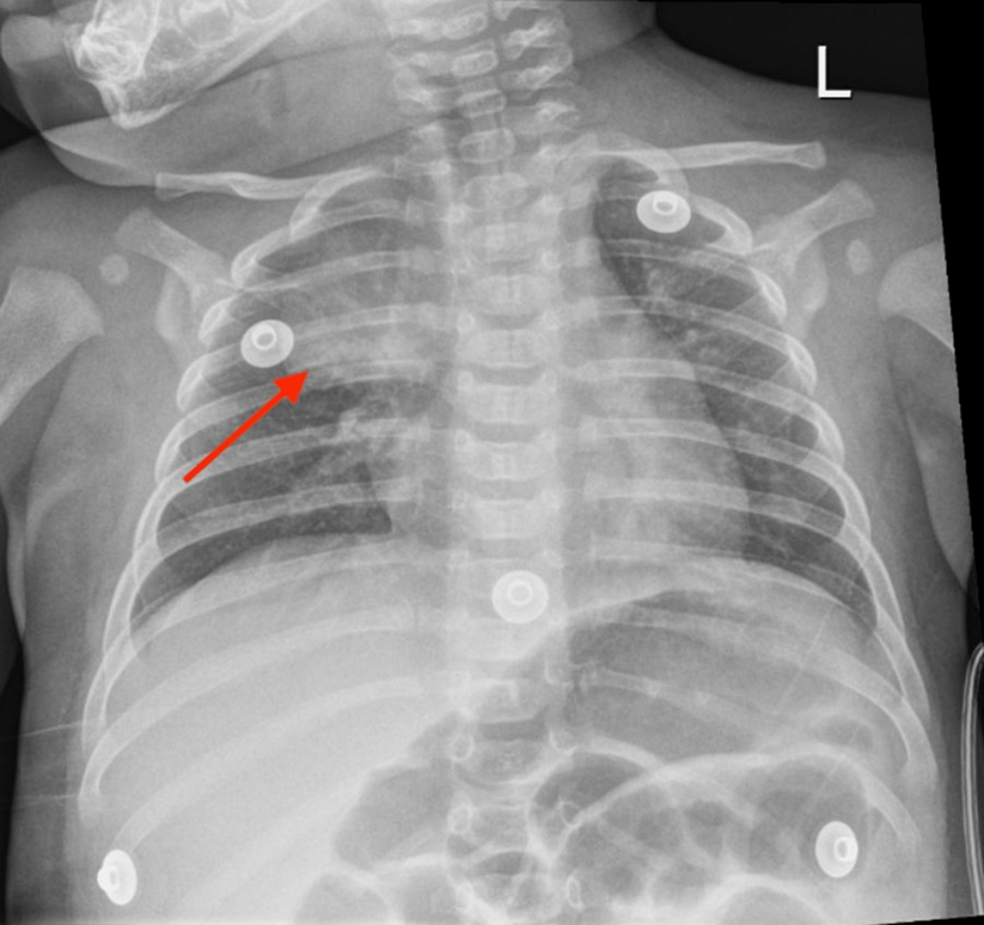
Chest radiograph showing focal right upper lobe pneumonia (arrow)
